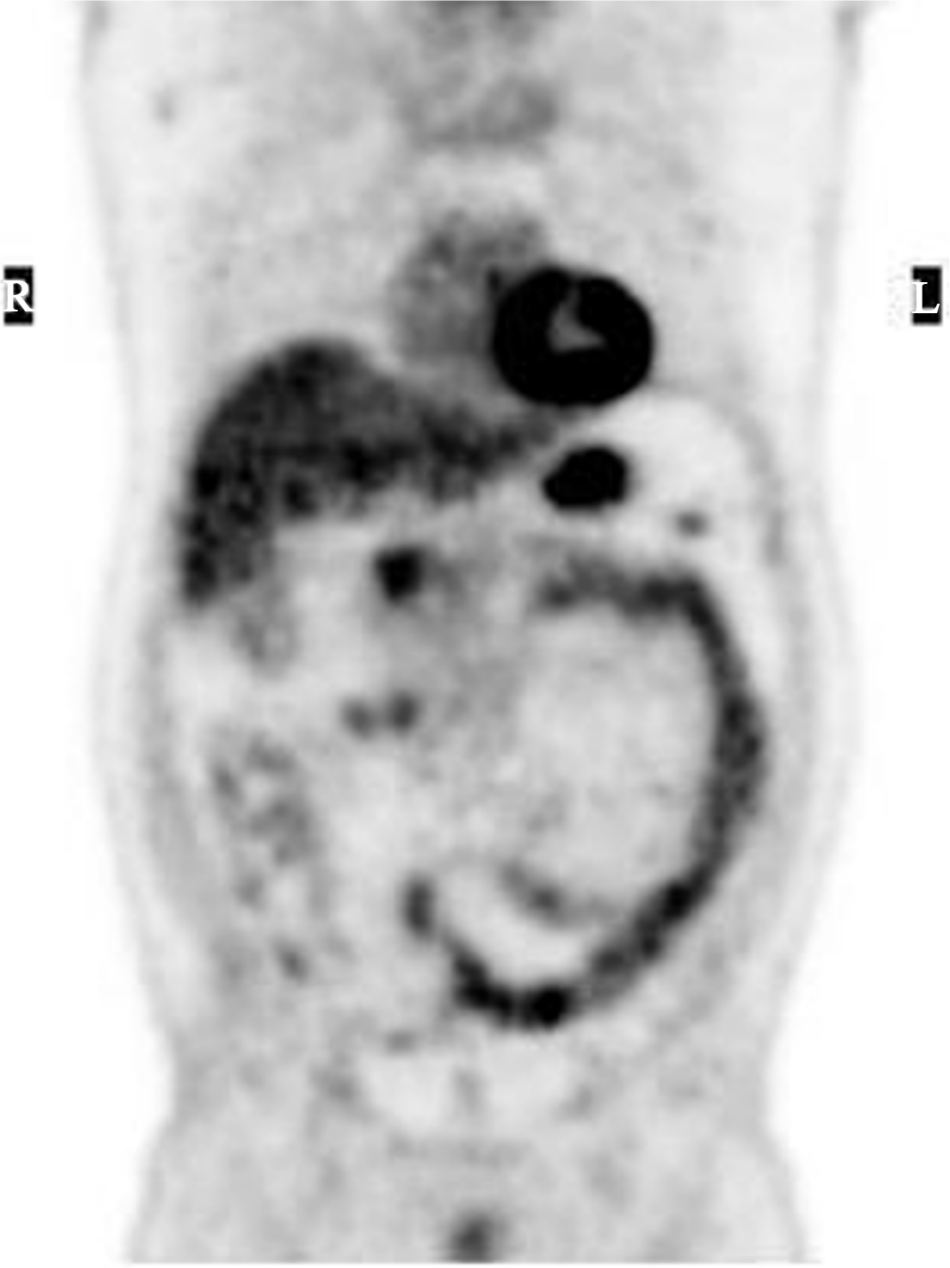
Image clearly showing ^18^FDG the in the descending colon (DC) and the sigmoid and rectum (S&R). The outline of the caecum and ascending colon (C&AC) can also be clearly seen. Note that only the left hand end (right hand end on the image) of the transverse colon (TC) is clearly visible. The transverse colon (TC) is approximately horizontal and extends from just below the lowest point in the liver (which can be clearly seen) to the top of the descending colon.
